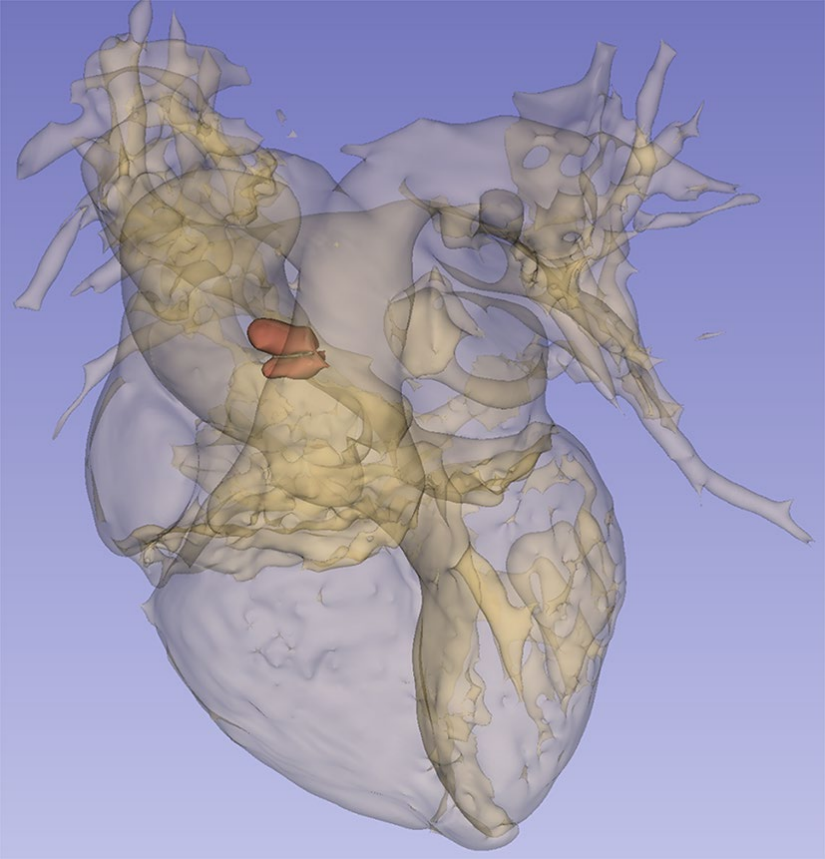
3D reconstruction based on coronary CTA revealing the presence of a large thrombus in the proximal portion of the ascending aorta, measuring 1.5 × 1.1 × 0.9 cm. Red structure indicates the thrombus
